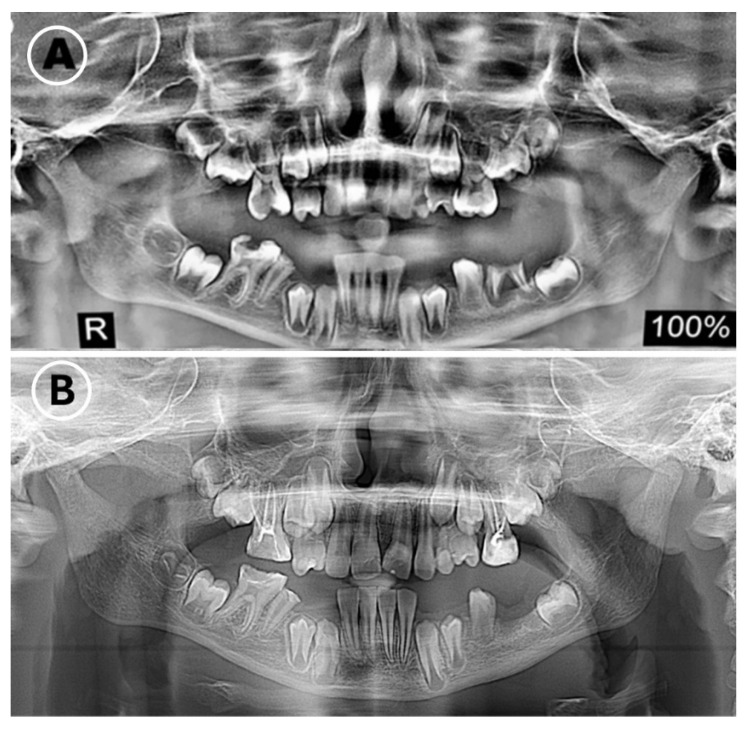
Pre-and post-operative panoramic radiographs showing multiple carious teeth and chronically infected lower left first molar remaining roots (A), and the treatment performed on all affected teeth (B).
